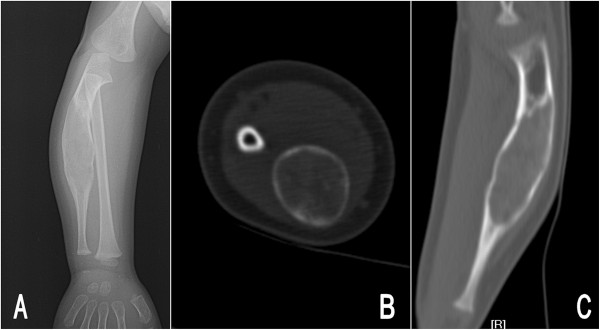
Preoperative images of the right forearm in patient 1. Posteroanterior radiograph (A) showed a fusiform-shaped, expansile ground-glass lesion in the ulna. Axial plane (B) and coronal reconstructed computed tomography (C) revealed the cortex was thinning and the area of lesion was homogeneous, with an average computed tomography value of 123 Hu.
